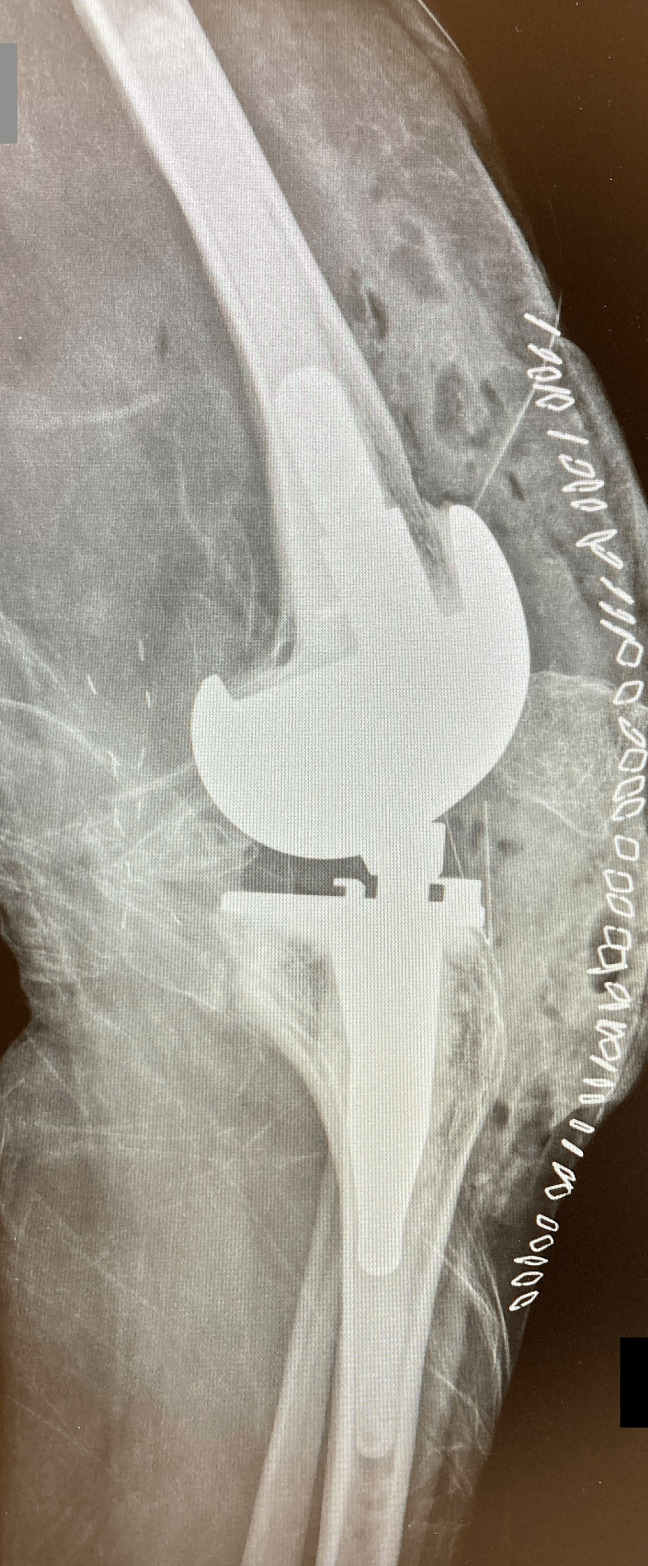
Postoperative lateral knee radiograph showing the cemented rotating-hinged total knee arthroplasty.
